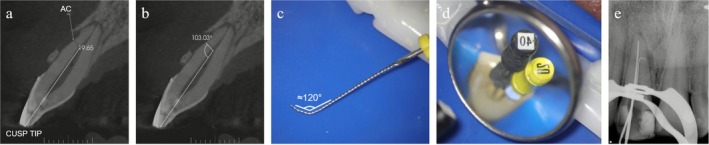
Accessory canal negotiation technique. (a) Measurement of the distance from the accessory canal to the incisal edge; (b) measurement of the angle formed between the accessory and main canal; (c) pre‐curved instrument; (d) instruments inserted into the main and accessory canal; (e) periapical radiograph after accessing the accessory canal.
